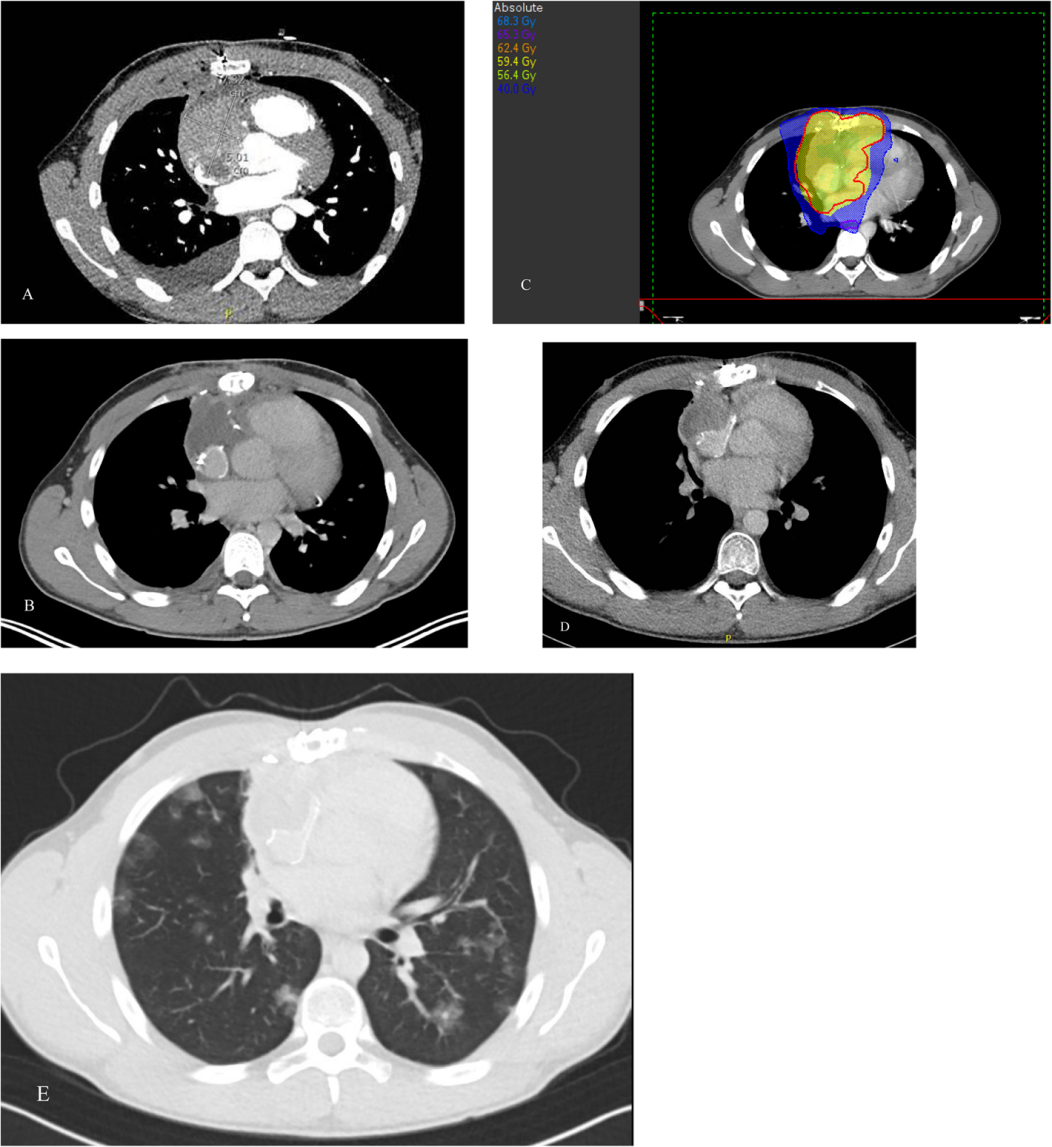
a. CT shows an inhomogenous mass located in the right atrium, expanding towards the pericardium and the anterior Mediastrium, with ingrowth into the superior vena cava. b. Scan made 3 weeks after surgery. c Radiotheraphy planning in transversal view. The red colored line represents the planning target volume. d. No evidence of disease 1 year after diagnosis. E. One year after finishing treatment CT shows local recurrence and mestastasis
